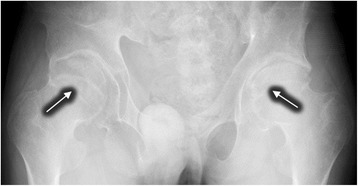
The plain film showed hip osteoarthritis with narrowing joint space, incomplete articular surface and swelling joint capsule (*Arrows*)
